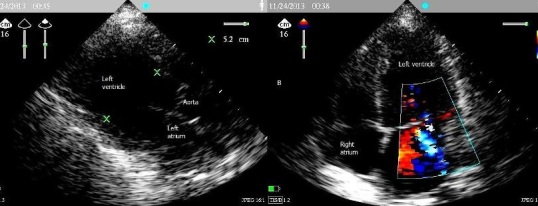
A) 2D echocardiography parasternal long axis showing a left ventricular dilatation in diastole with 3.25 cm/m^2^ (calculated body surface area according to the Mosteller formula: S = √ (L ×M /3600); S is the body surface area in m^2^, L is the size in cm, M is the mass in kg); B) apical four-chamber view with color Doppler showing mitral regurgitation grade I to II
